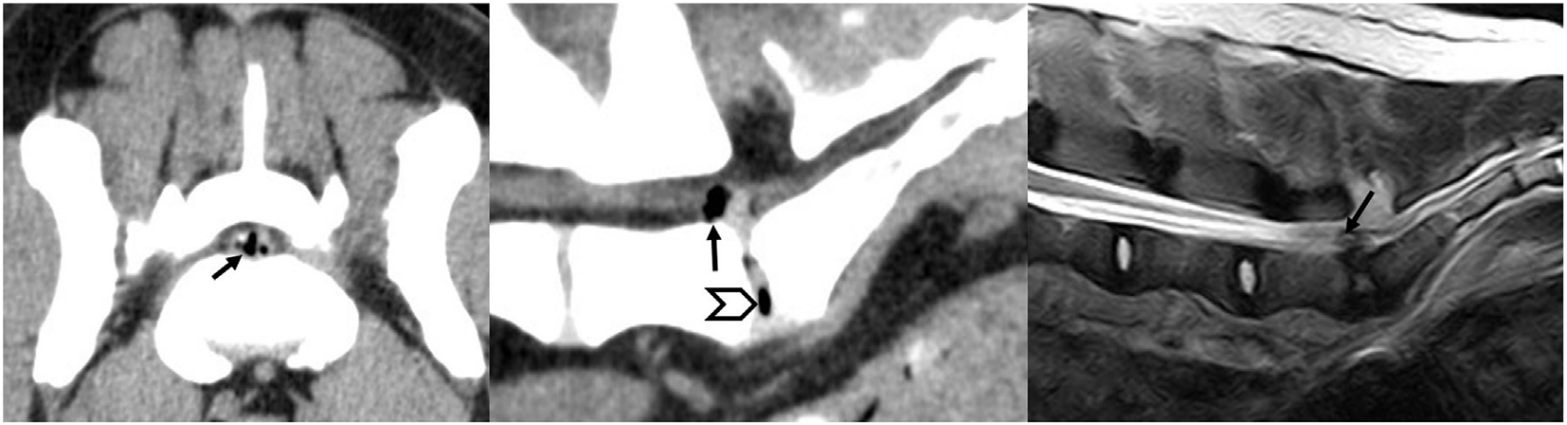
Transverse computerized tomography (CT) and sagittal CT and magnetic resonance imaging images of case 1 showing the symptomatic compression of the cauda equina by a gas-containing disc protrusion (arrow) that appeared 3 years after the first surgical procedure done to remove the intervertebral disc protrusion. The vacuum phenomenon is visible on the sagittal CT image (arrow head).
